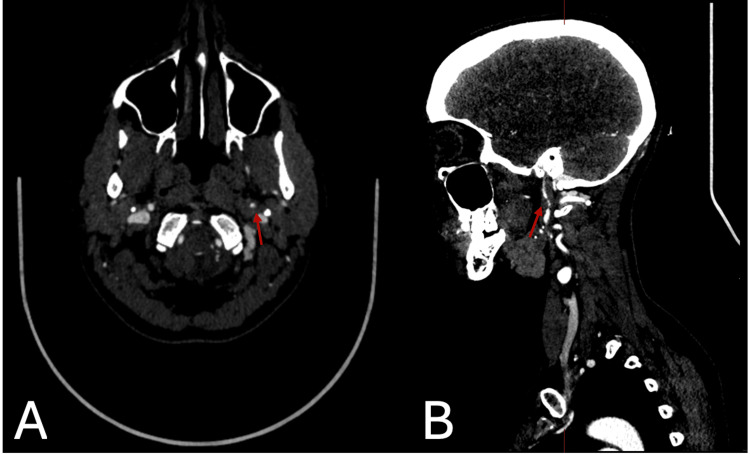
Axial (A) and sagittal (B) CT imaging demonstrating circumferential narrowing of the left internal carotid artery (ICA), with increased density in the vessel wall consistent with an intramural haematoma.
